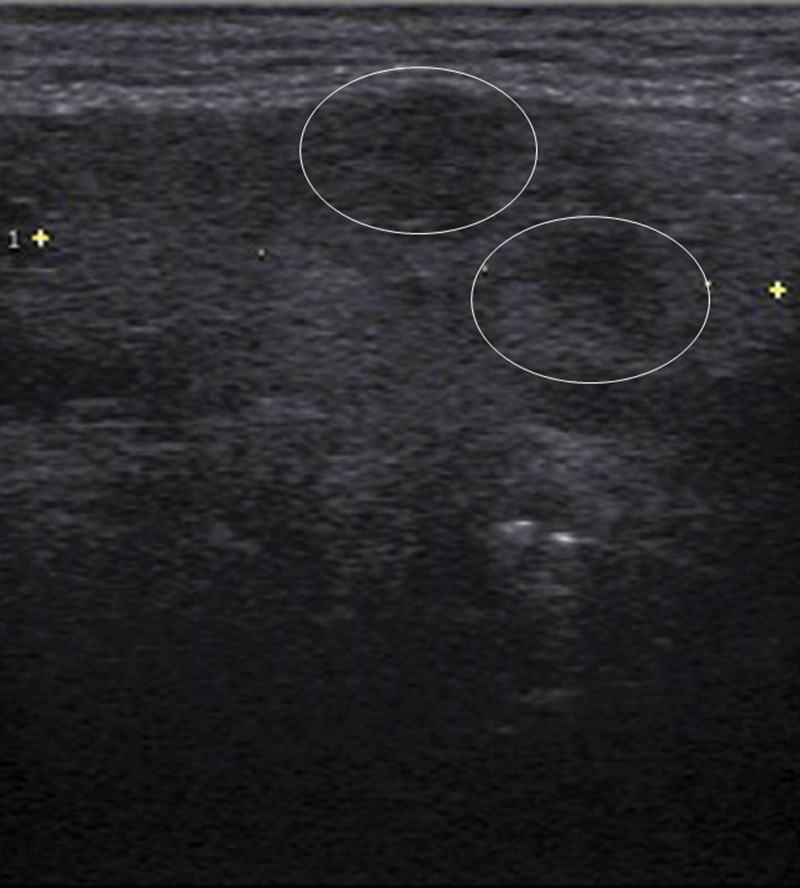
Submandibular gland ultrasonography before treatment revealing coarse hypoechoic areas (circle).
